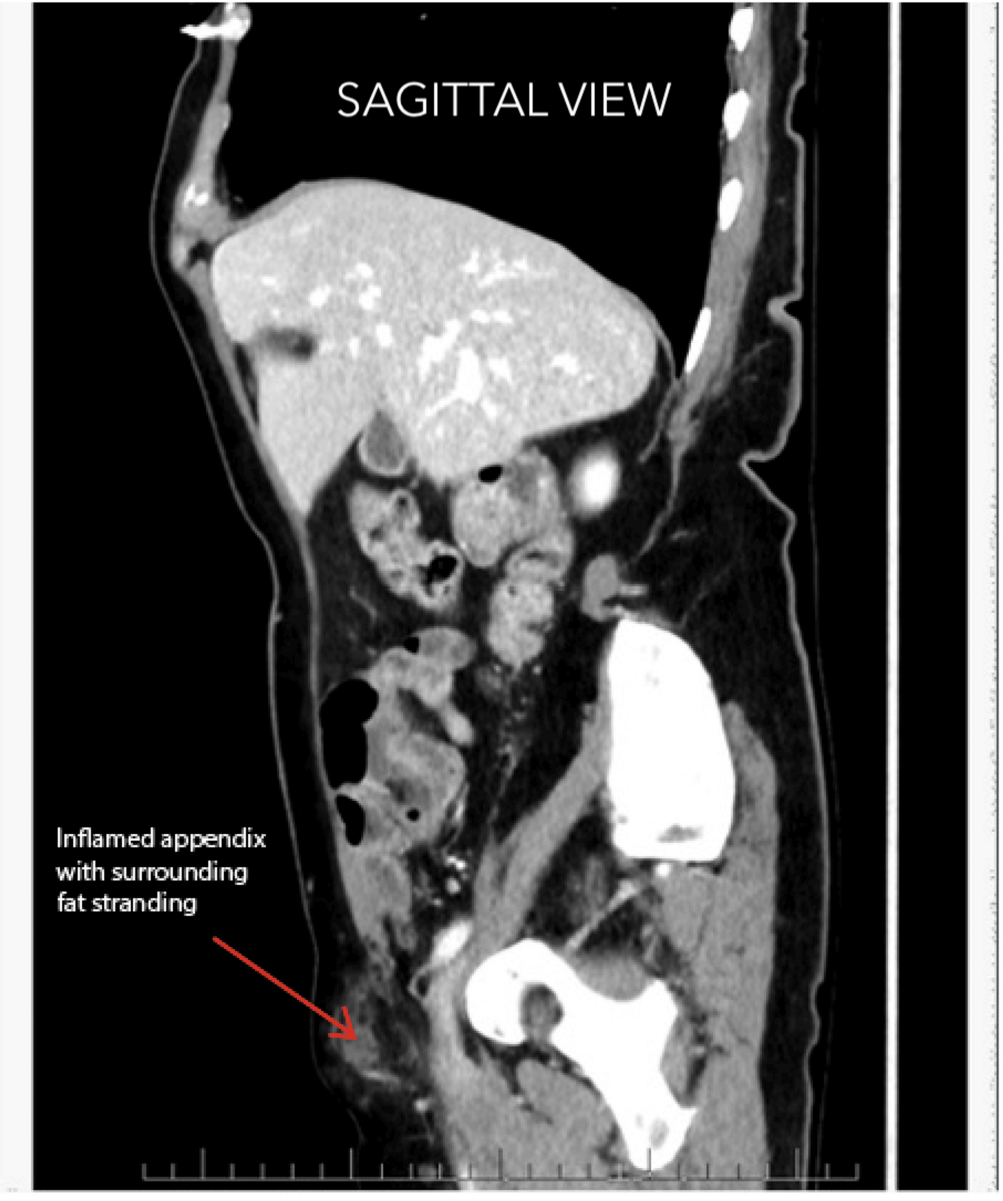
CT sagittal view showing inflamed appendix and fat stranding in De Garengeot hernia
